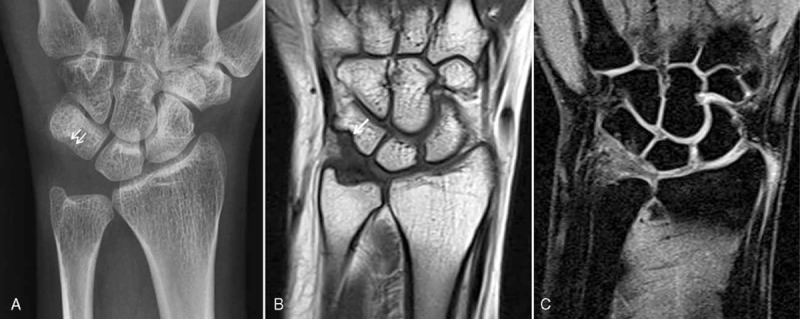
A, Anteroposterior radiograph shows no more progress of bone erosion in the triquetrum (arrows) in the 24-month follow-up magnetic resonance image of the left wrist after excision. B, Coronal T1-weighted image shows no evidence of nodular lesion to suspect a recurred mass. Note the erosion of the triquetrum from the compression of the previous mass (arrow). C, Coronal fat-suppressed T2-weighted image reveals postoperative fibrotic change in the triangular fibrocartilage complex. There is no intra-articular of the left wrist at 24-month follow-up, showing the complete lack of recurrence of the giant cell tumor.
